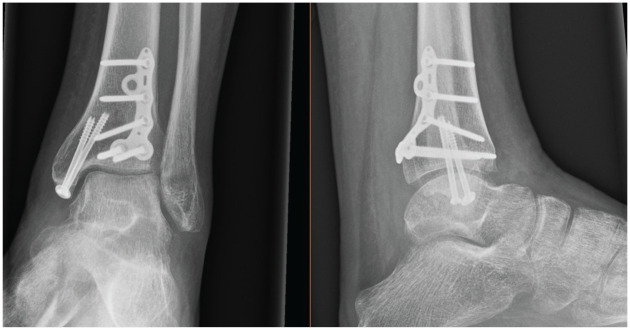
Radiograph showing (Volition) type IIA (posterolateral tibial plate) in situ.
